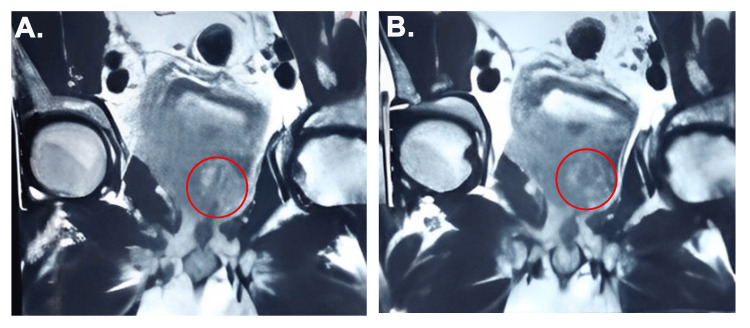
(A-B) Multiparametric MRI T2-weighted MRI reveals intermediate signal intensity suggestive of irregular mass in the prostate involving the left pelvic wall and abutting the wall of left external iliac vessels (red circle).
